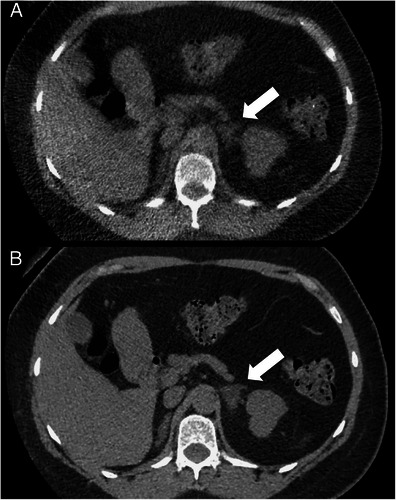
A 40-year-old female patient (BMI = 29.8) with suspected renal calculi. a Axial low-dose (0.45-mSv) 2-mm non-contrast CT image reconstructed with 70 % ASiR and b axial conventional dose (7 mSv) 2-mm non-contrast CT image reconstructed with 40 % ASiR showing a 3.2-cm low-density left adrenal mass consistent with a benign adenoma (*arrow*). This left adrenal mass was missed when viewed on the low-dose non-contrast CT images
